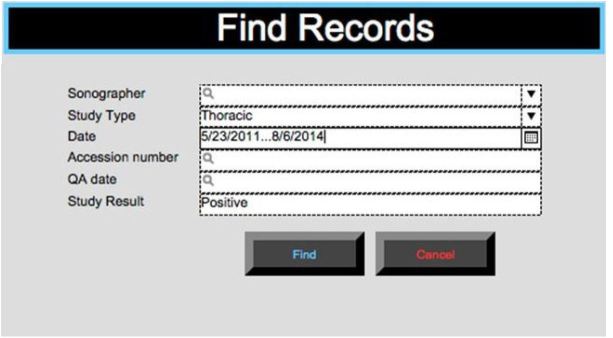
Example of the thoracic ultrasound reporting template in the emergency department quality assurance database (Filemaker Pro, Santa Clara, CA).
